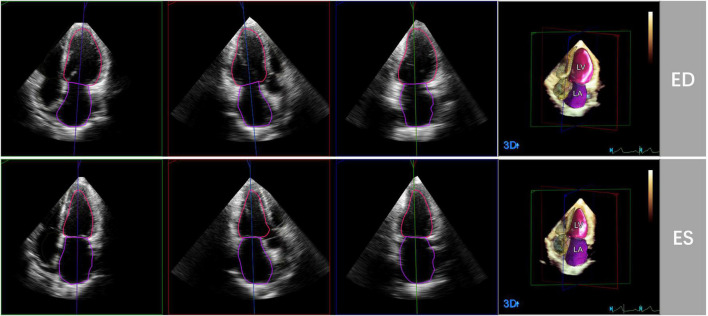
Representative case of automated 3D echocardiographic analysis for left heart chamber quantification. Left heart chambers’ endocardial borders were automatically detected by the Heart Model software at end-diastole (ED) and end-systole (ES) in apical four-, three-, and two chamber sections.
